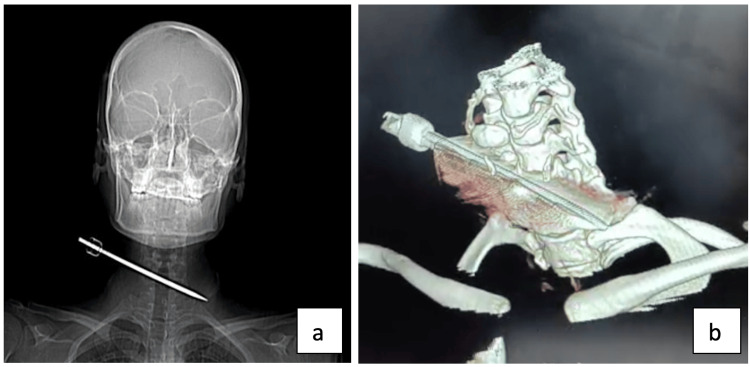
CECT neck depicting the foreign body: (a) coronal view and (b) 3D reconstruction CECT: Contrast-enhanced computed tomography.
